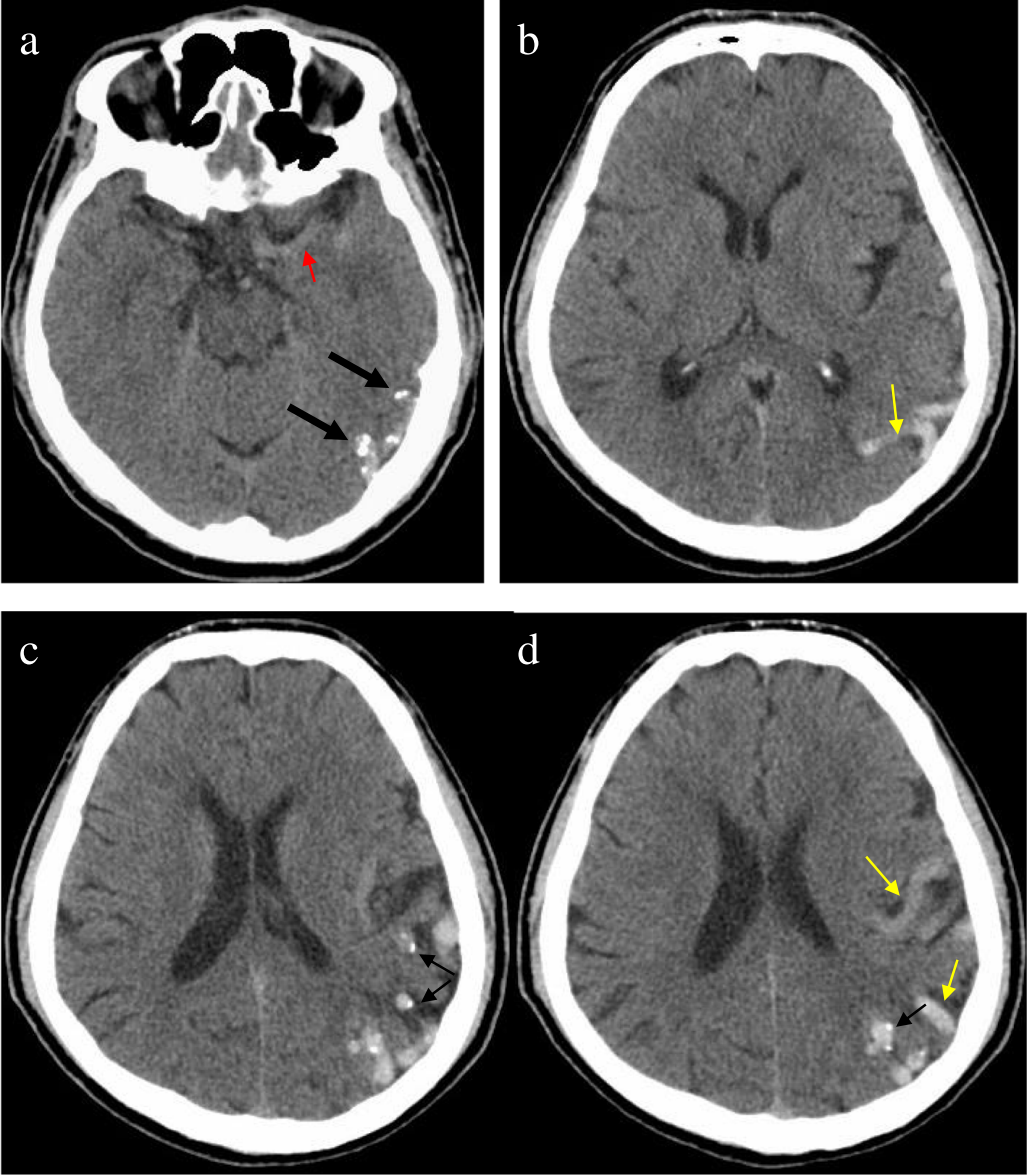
Head CT scan on admission showed presence of abnormal tortuous and dilated vessel in the left temporal and parietal lobe (yellow arrow), multiple punctuate calcification (thin black arrow) and nodular calcification (thick black arrow) and the tortuous and enlarged left MCA (red arrow)
